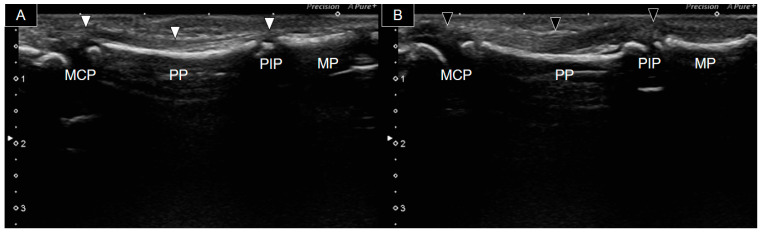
Ultrasound image of the palmar side of the second right (A) and left (B) fingers. The right flexor tendon (white arrowheads) was significantly thinner than the left one (black arrowheads) and was not visible over the right middle phalanx. MCP, metacarpophalangeal joint; PP, proximal phalanx; PIP, proximal phalangeal joint; MP, middle phalanx.
